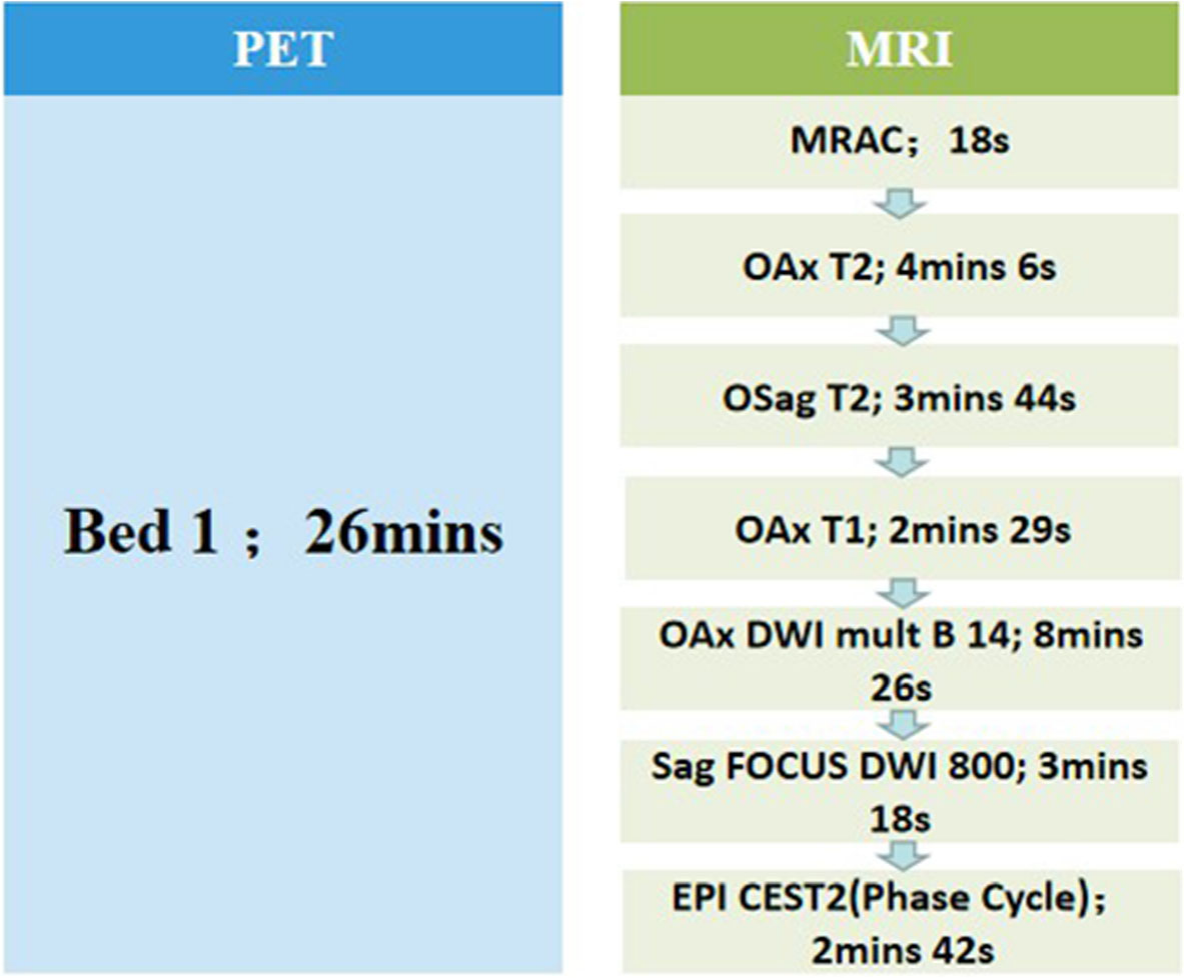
PET and MRI scan at the same time, each sequence of MRI is performed in sequence, the total time is about 26 min
